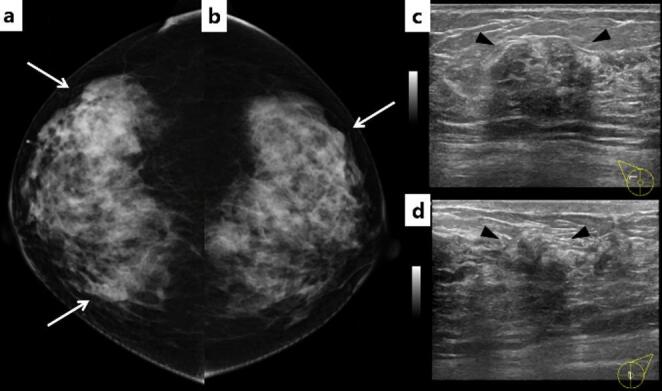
Diabetic mastopathy. a–b Mammography, c–d ultrasound. A woman in her 60s with type 2 DM underwent dialysis for diabetic kidney disease. Mammography (a–b) shows bilateral asymmetric local densities (arrows). Ultrasound (c–d) shows hypoechoic lesions with posterior acoustic shadowing (arrowhead). Biopsy revealed diabetic mastopathy, and ultrasound and mammography showed no gross interval changes in the imaging findings over time (not shown). Abbreviation: DM, diabetes mellitus
